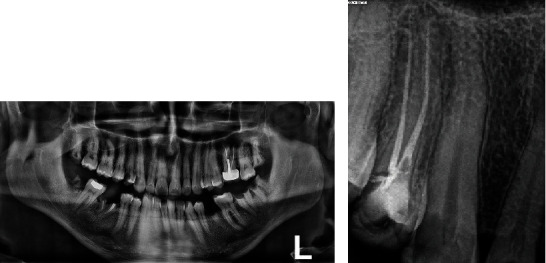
Pretreatment. (a) Panoramic and (b) periapical radiographs.
